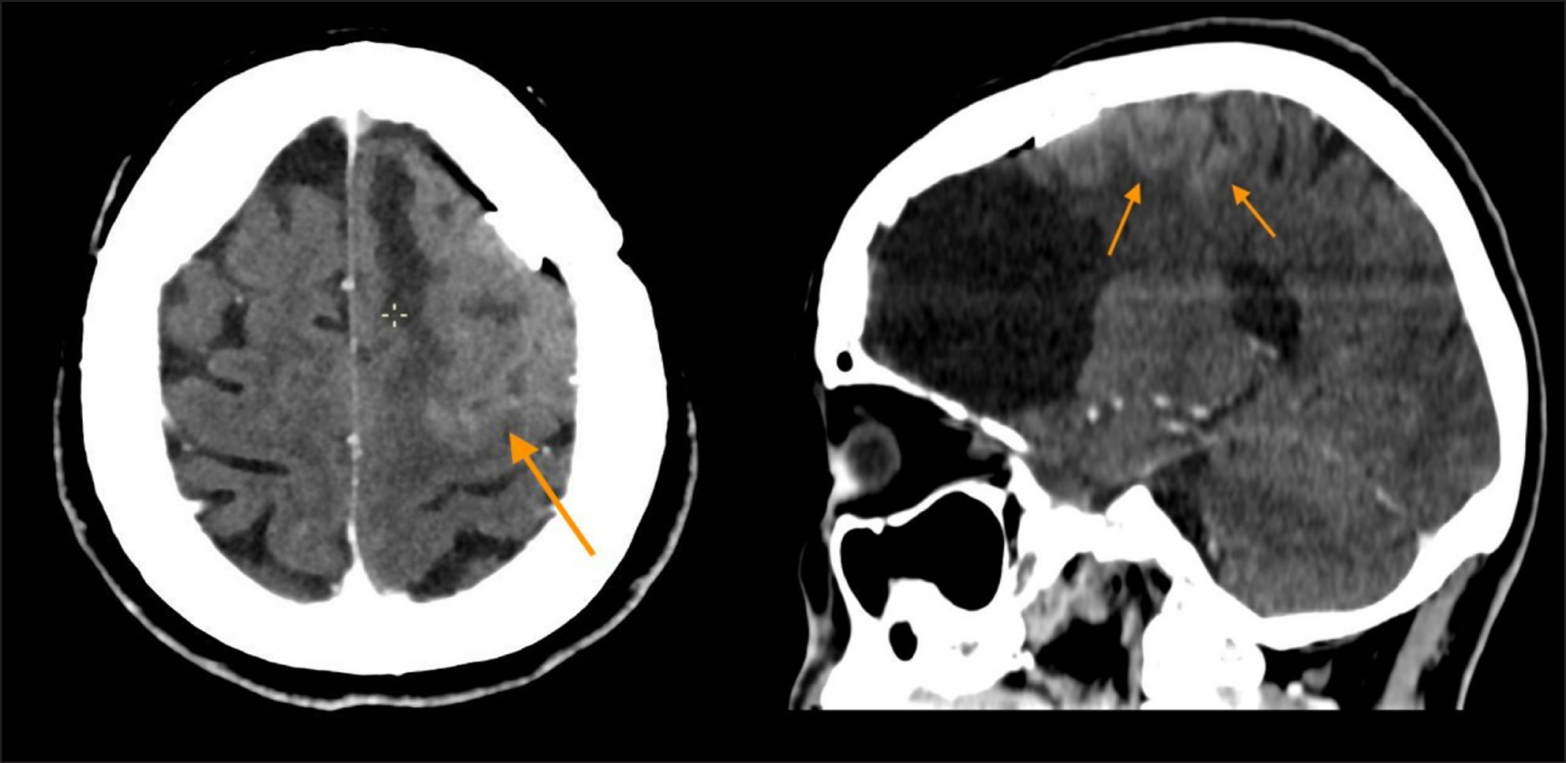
Axial and sagittal CT section shows corticopial enhancement in the left posterior frontal lobe surrounding the resection cavity.
